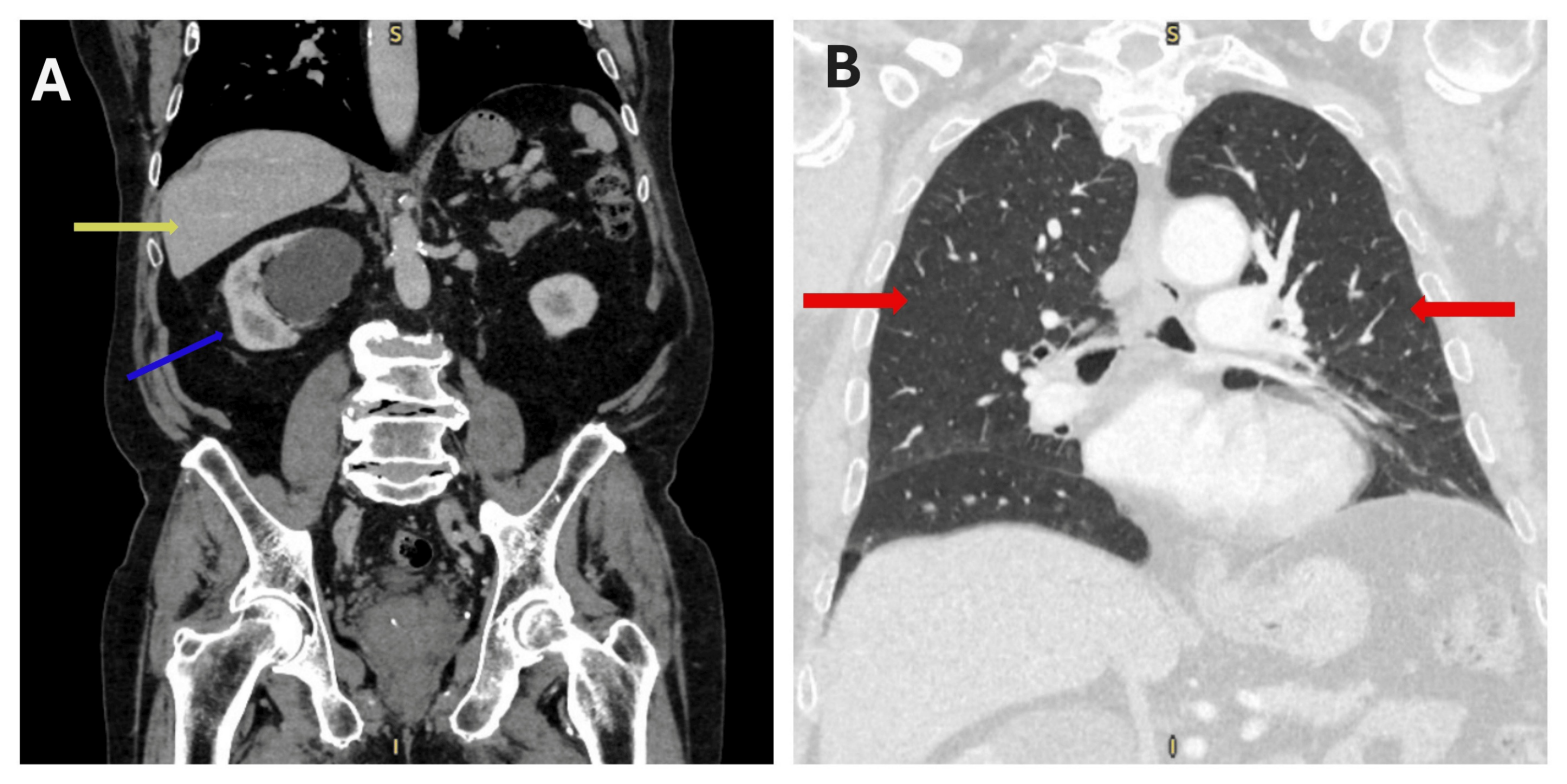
Postoperative staging CT scan of the thorax, abdomen, and pelvis with contrast. (A) Abdomen and pelvis showing no metastasis. The yellow arrow points to the liver and the blue arrow points to the right kidney with an incidental simple cyst. (B) Chest scan showing no metastasis (red arrows point to the lungs).
